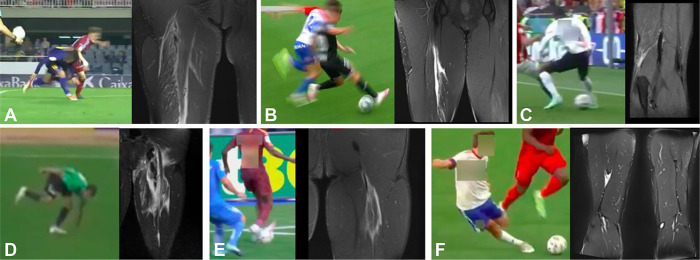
Assumed injury frames and MRI findings in mixed-type injuries. A, Case 1, proximal BF injury. B, Case 5, mid-thigh SM injury. C, Case 6, distal BF avulsion. D, Case 7, proximal BF + ST avulsion. E, Case 9, proximal BF injury. F, Case 10, distal ST injury of the MTJ. MTJ, myotendinous junction
